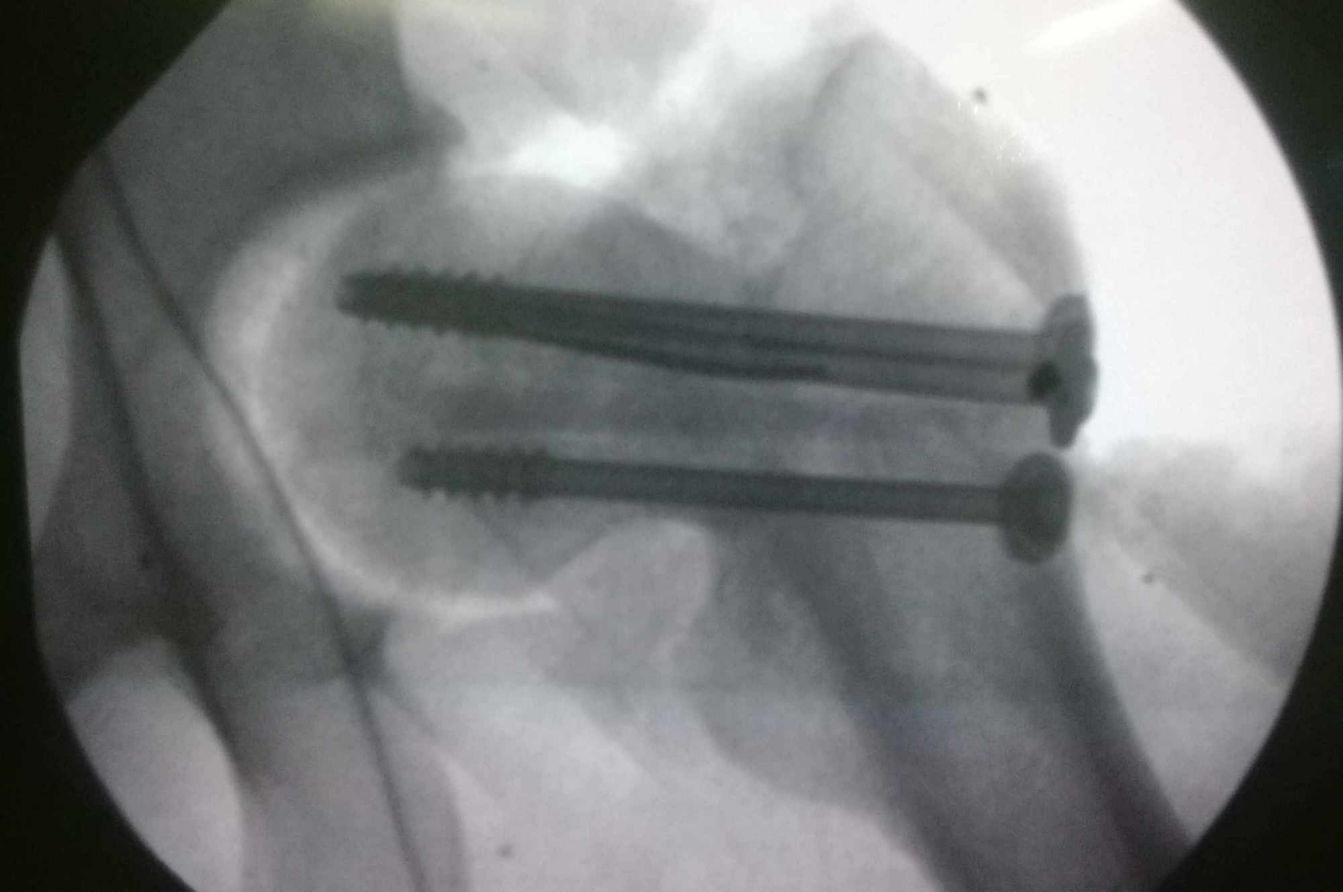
Intra-operative radiograph of patient B showing the screws and central fibula.
